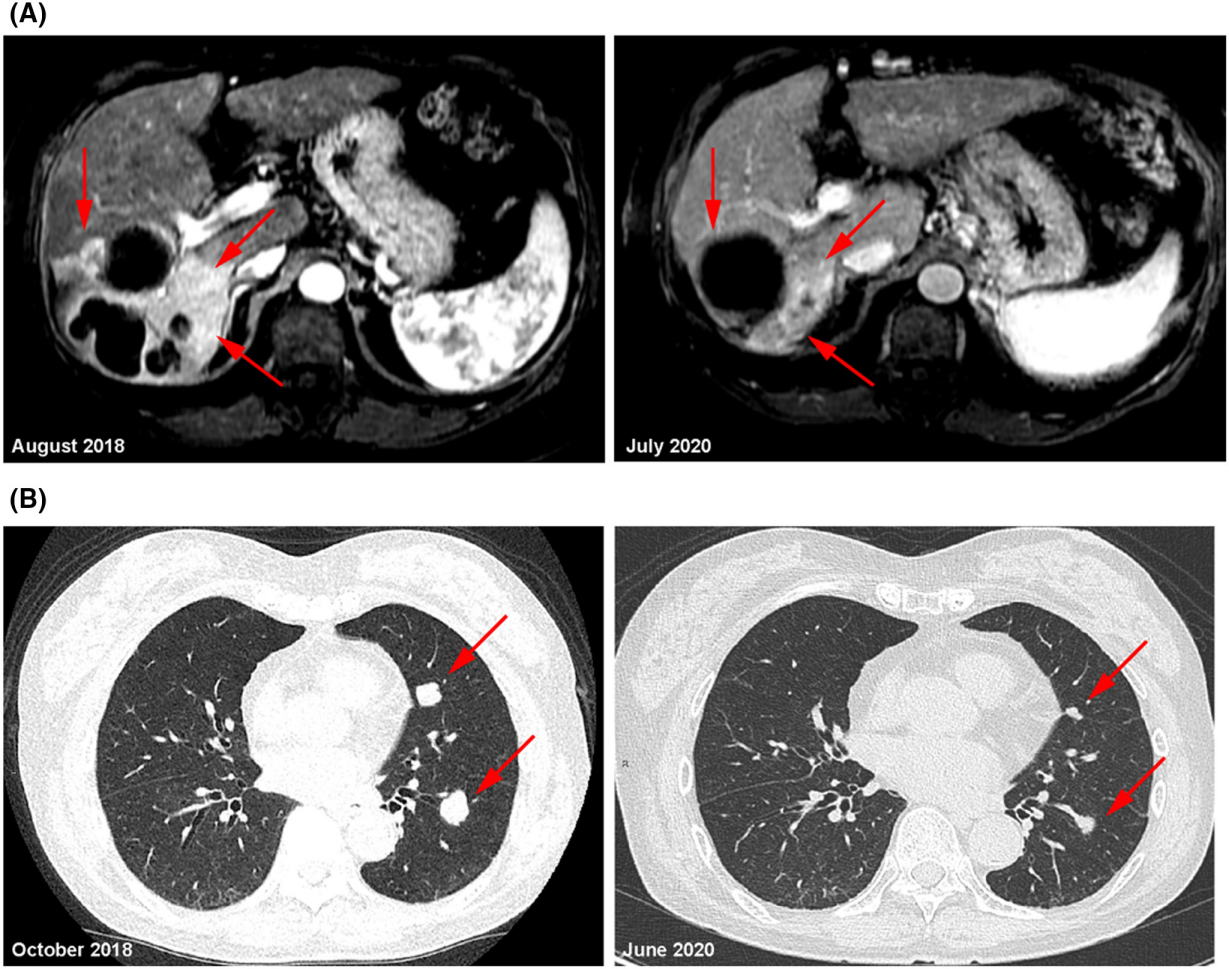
Imaging of two patients with partial radiographic responses. (A) Metastatic liver disease in a patient receiving 35 AutEMdev EMF exposure procedures as monotherapy (magnetic resonance imaging). (B) Metastatic lung disease in a patient receiving 31 AutEMdev EMF exposure procedures in combination with first‐line lenvatinib (computed tomography). Red arrows indicate tumors. Abbreviations: AutEMdev indicates Autem electromagnetic device; EMF, electromagnetic field.
